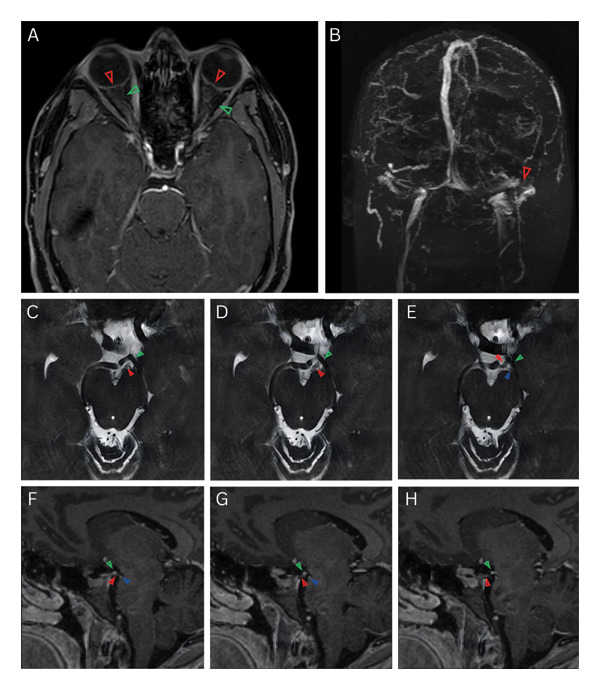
(A) Axial contrast‐enhanced T1‐weighted magnetic resonance imaging (MRI) of the orbits (with orbital fat suppression; 0.9‐mm slice thickness) demonstrating bilateral optic nerve head cupping (red arrows) and tortuosity of the optic nerves (green arrows), with left more tortuous than right. (B) Magnetic resonance venography of the brain revealing a left transverse sinus stenosis (red arrow). (C–E) Axial fast imaging employing steady‐state acquisition (FIESTA) MRI of the brain (1.4‐mm slice thickness) delineating the course of the left oculomotor nerve (red arrow) under the left posterior cerebral artery (PCA; green arrow) and above the left superior cerebellar artery (SCA; blue arrow). (F–H) Sagittal contrast‐enhanced T1‐weighted MRI of the brain (1‐mm slice thickness) showing the course of the left oculomotor nerve between the left PCA (green arrow) and SCA (blue arrow).
